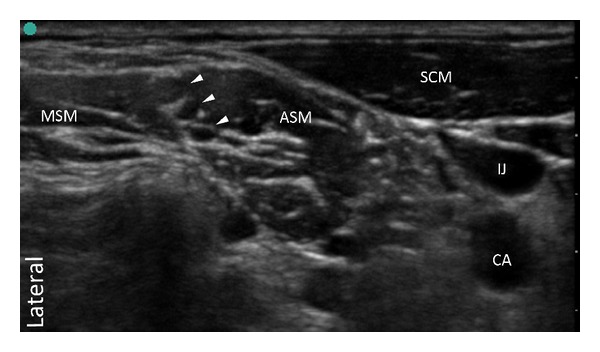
Transverse ultrasound image of interscalene brachial plexus. Arrowheads outline brachial plexus roots within the interscalene groove. SCM: sternocleidomastoid muscle, IJ: internal jugular vein, CA: carotid artery, ASM: anterior scalene muscle, MSM: middle scalene muscle.
